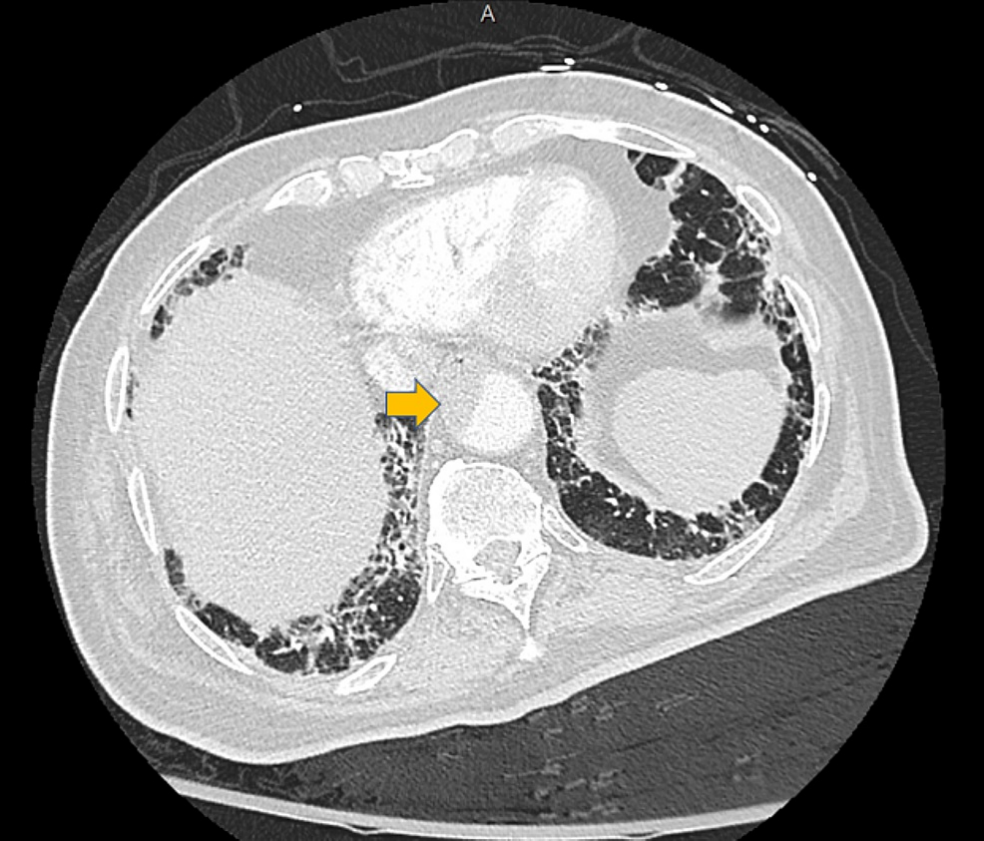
Computed tomography with intravenous contrast of chest Focal 6.2 cm intraluminal thrombus narrowing 40% of the aorta at the level of the diaphragmatic hiatus
